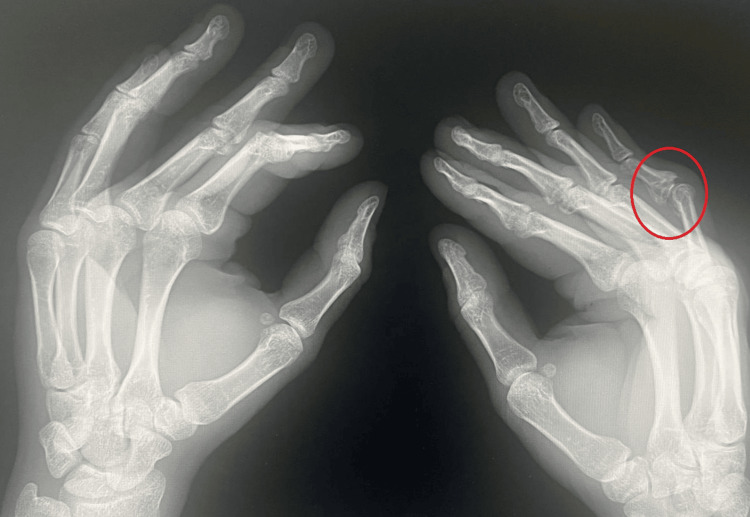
X-ray of both hands before surgery in lateral projection Red circle indicates the proximal interphalangeal joint.
